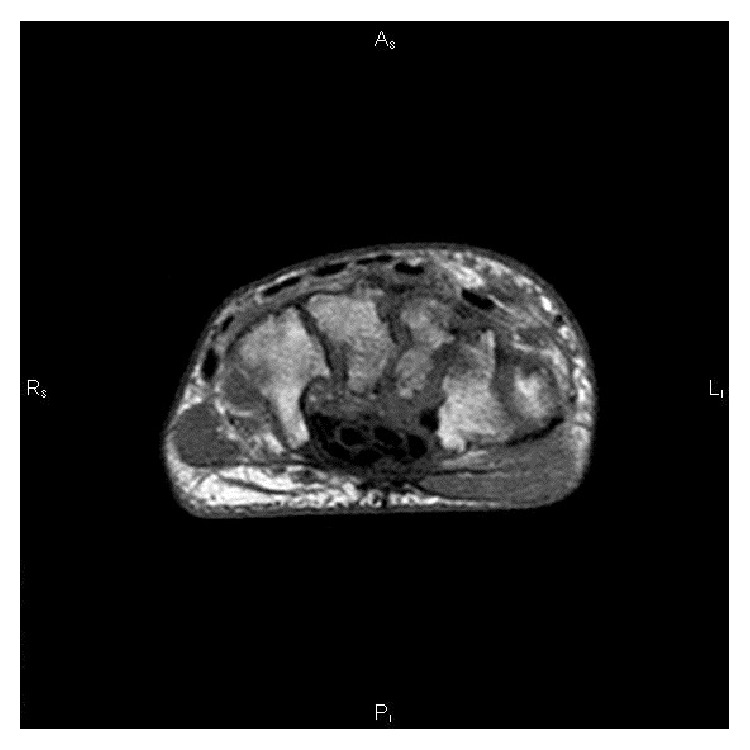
Magnetic resonance imaging of the left wrist after three months of specific antibiotic therapy versus* Brucella *spp. and* Mycobacterium bovis*. The picture shows slight decrease of signal intensity of trabecular bone of radium and carpal bone and moderate reduction of edema extension in scaphoid and capitate bones.
